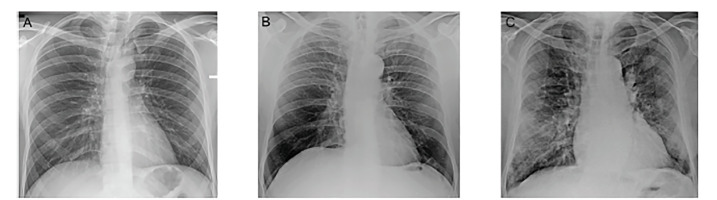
Radiographs of three different COVID-19 patients who presented with fever and cough at different time frames from disease onset. (a) A 32-year-old man who presented one day after symptom onset. Radiograph assessment of lung edema (RALE) scoring: reader 1: 0; reader 2: 0. (b) A 64-year-old man who presented three days after symptom onset. RALE scoring: reader 1: 1; reader 2: 2. (c) A 73-year-old man who presented seven days after symptom onset. RALE scoring: reader 1: 6; reader 2: 6.
